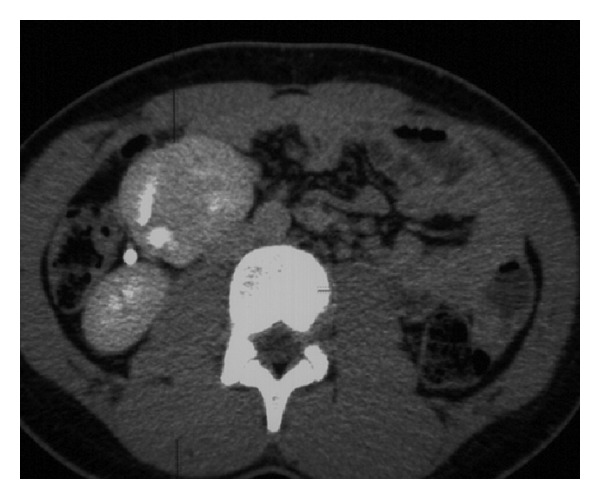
16-year-old adolescent male with left-to-right crossed renal ectopia. Axial CT scan with intravenous contrast-delayed phase demonstrates two normal enhancing kidneys on the right.
